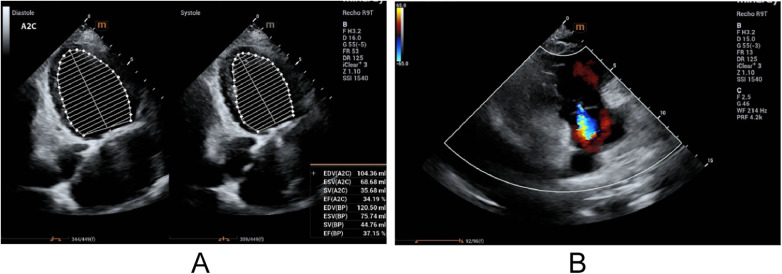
Echocardiographic assessment of left ventricular function and valvular regurgitation. (A) Two-chamber (A2C) views with speckle-tracking strain analysis for evaluation of myocardial deformation. (B) Corresponding color Doppler image demonstrating regurgitant flow across the cardiac valve.
